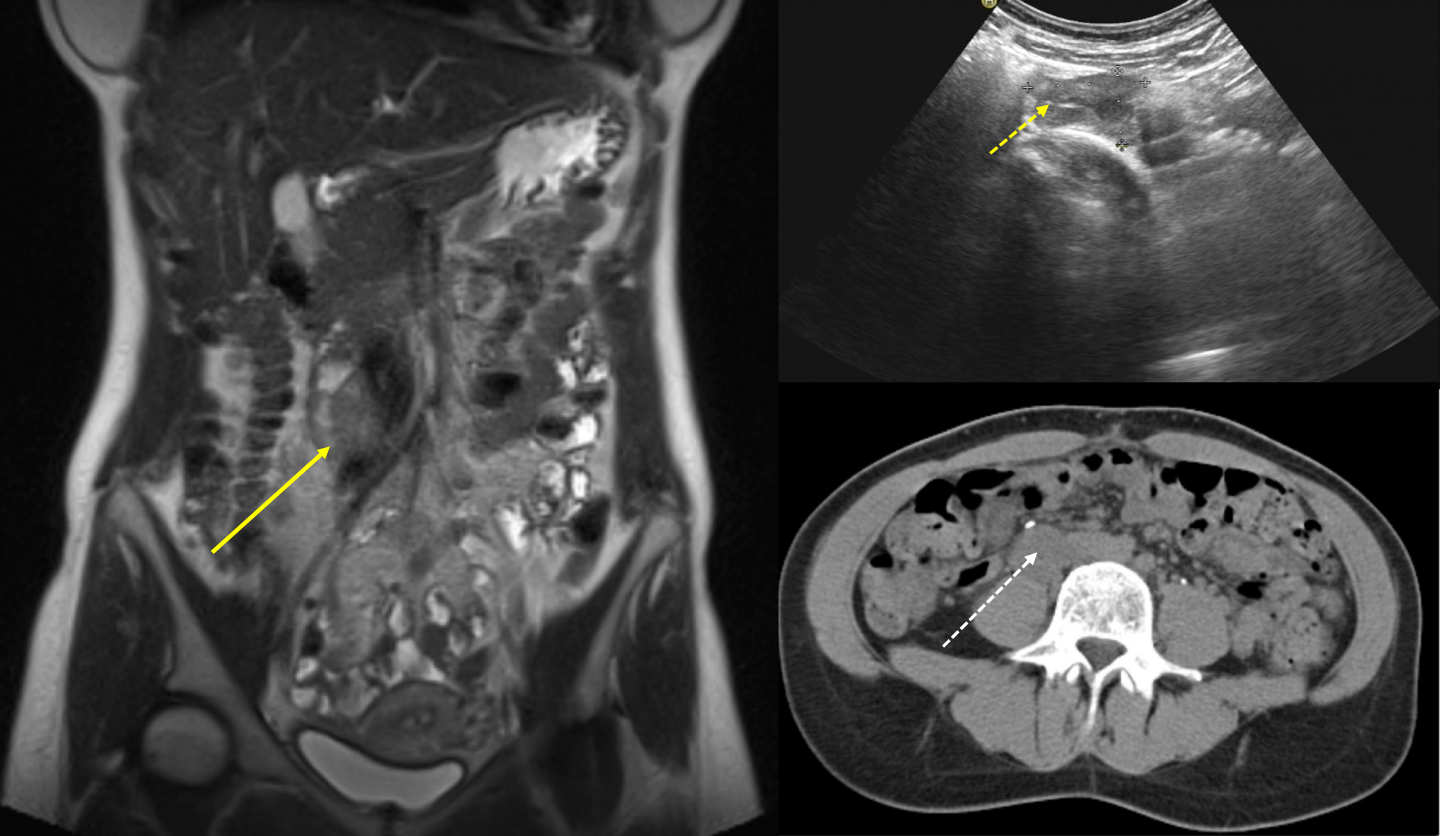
Preoperative coronal MRI showing a 68 × 35 × 28 mm solid mass anterior to the right psoas muscle (*yellow arrow*). The tumour was also detected by ultrasound and CT (*short yellow arrow*, *white arrow*)
